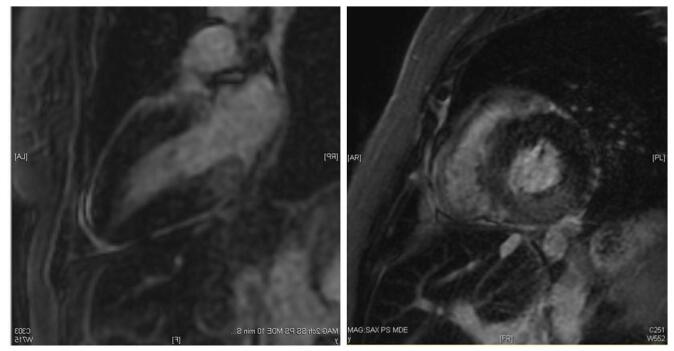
Cardiac MRI (Patient#2): Mid-myocardial LGE at the basal inferior/inferolateral walls.
